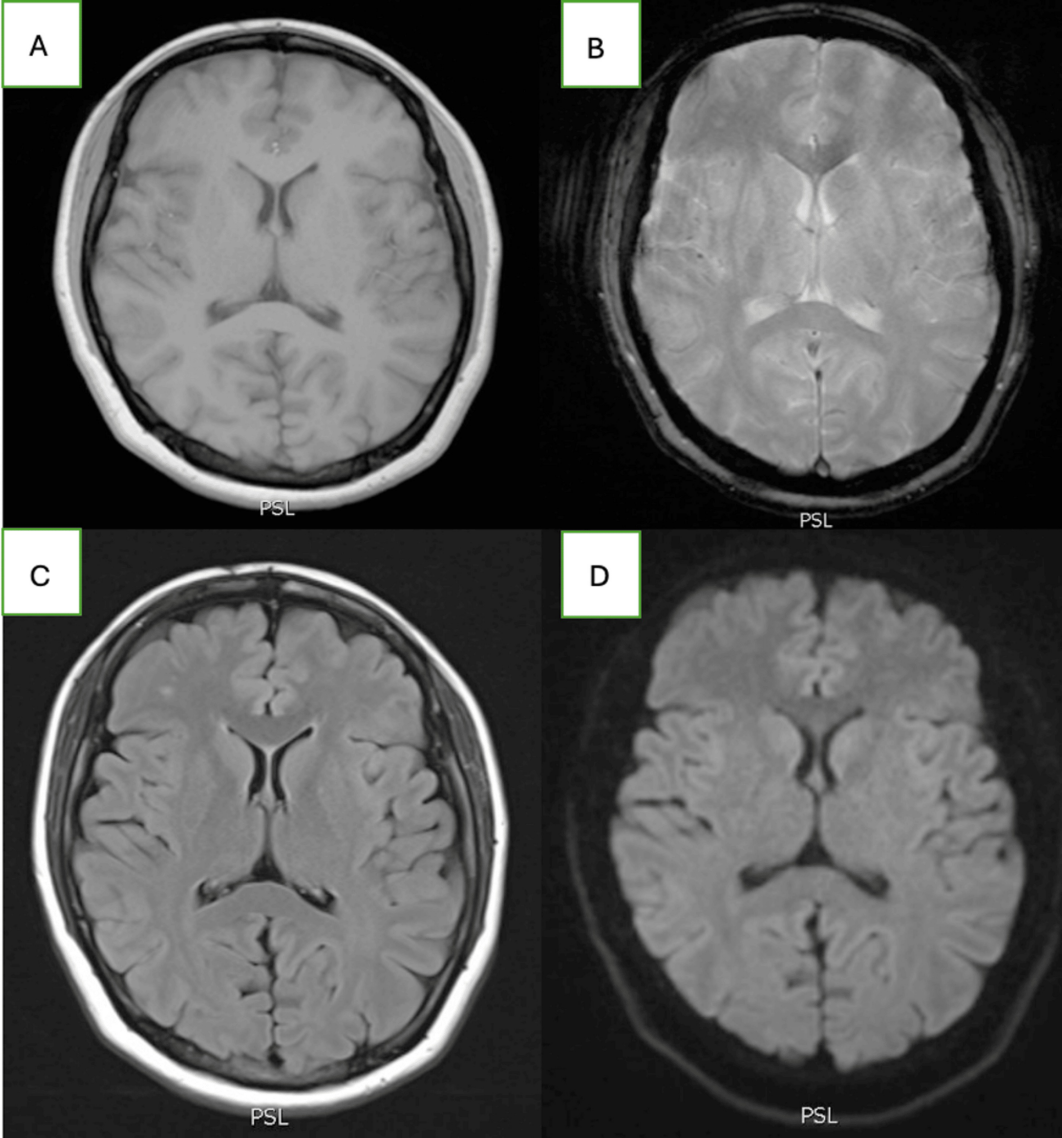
Normal MRI of the brain (A) Normal T1-weighted sequence, (B) Normal T2-weighted sequence, (C) Normal FLAIR sequence, (D) Normal diffusion-weighted imaging FLAIR: fluid-attenuated inversion recovery
